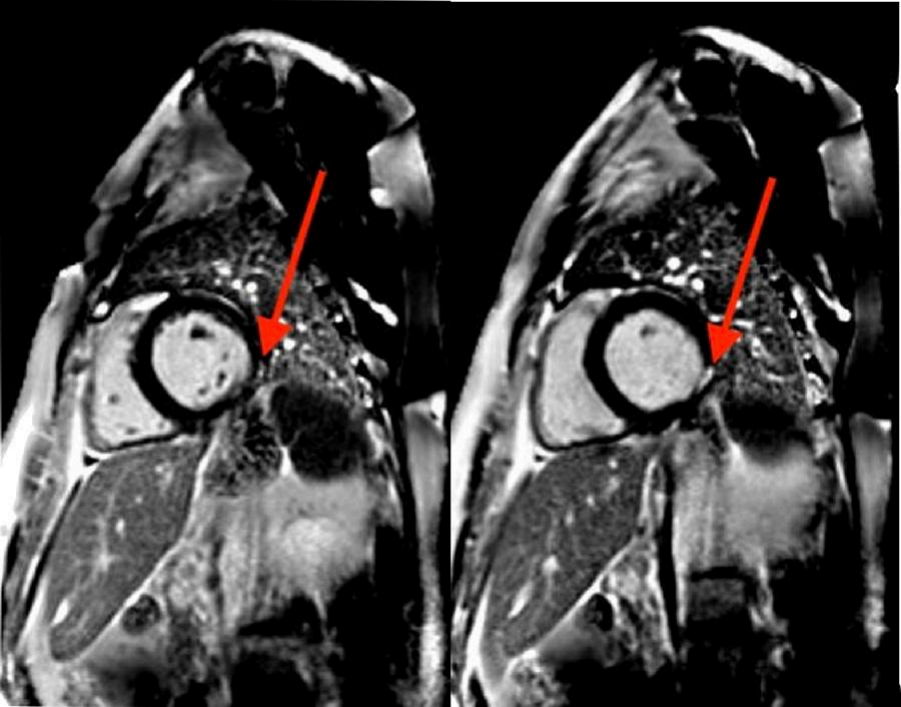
Cardiac magnetic resonance imaging. MRI short-axis views demonstrated late gadolinium enhancement of the basal inferolateral wall that was predominantly mid-myocardial. This finding can be seen in chronic myocarditis. T2 imaging result was negative.
